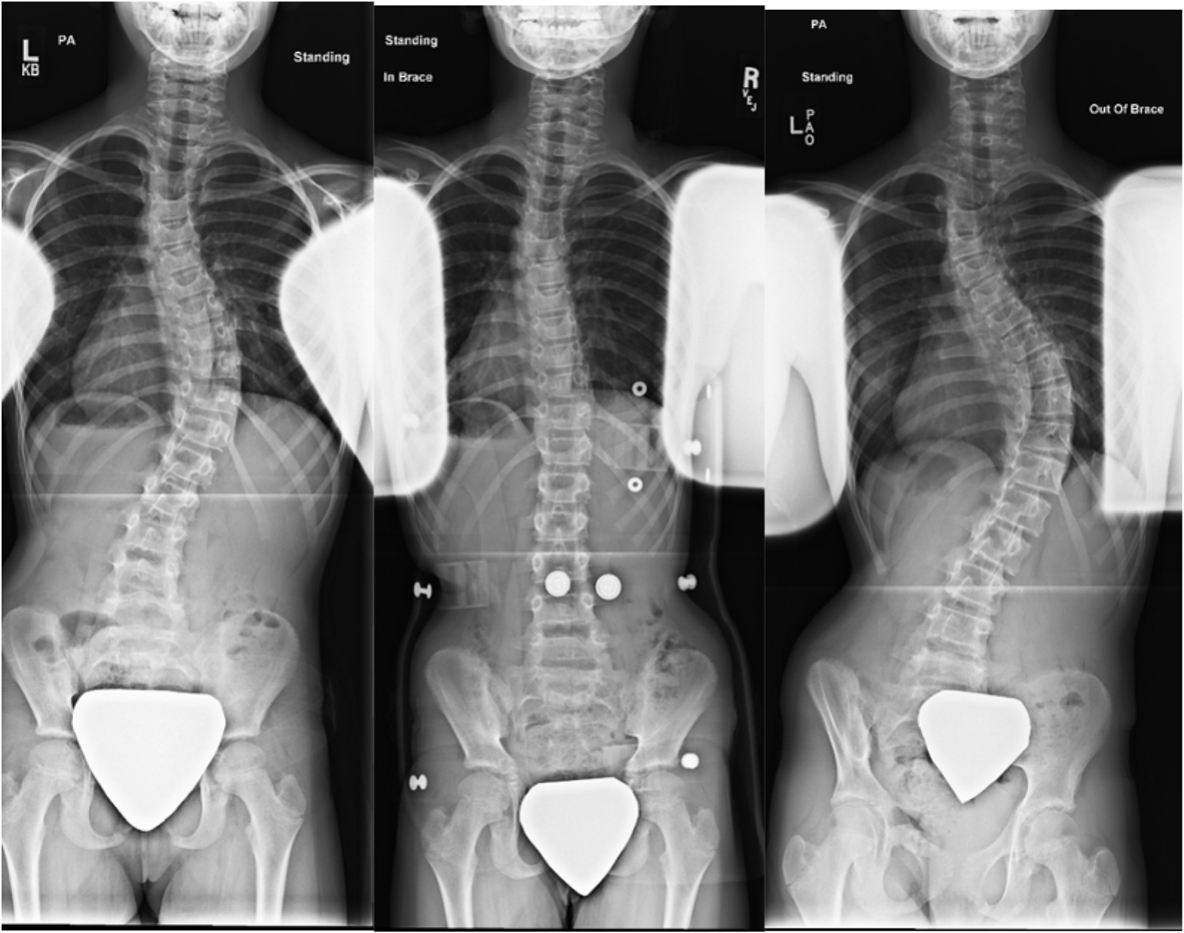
Patient example. This patient is a 10-year-old female who was Risser 0 with open tri-radiate cartilage at brace initiation. Her thoracic curve measured 44° initially (a). In her brace, her curve decreased to 9.9°, a 77.6% correction from her original curve (b). Thirteen months later, the patient was fitted for a second brace, but new in-brace x-rays were not taken. She was fitted for a third brace 15 months later without confirmation x-rays. This patient was compliant with bracewear; however, for 57.5% of her treatment time, she had an unknown in-brace correction. She ultimately discontinued brace use with a final curve of 63.8°, requiring surgery (c)
